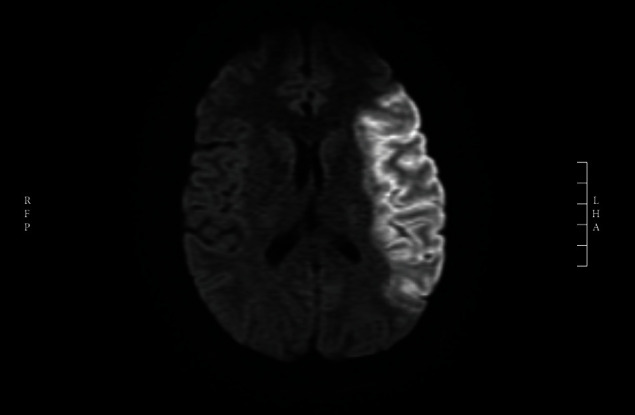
Diffusion-weighted brain magnetic resonance imaging (MRI) showing acute stroke in the left middle cerebral artery territory.
